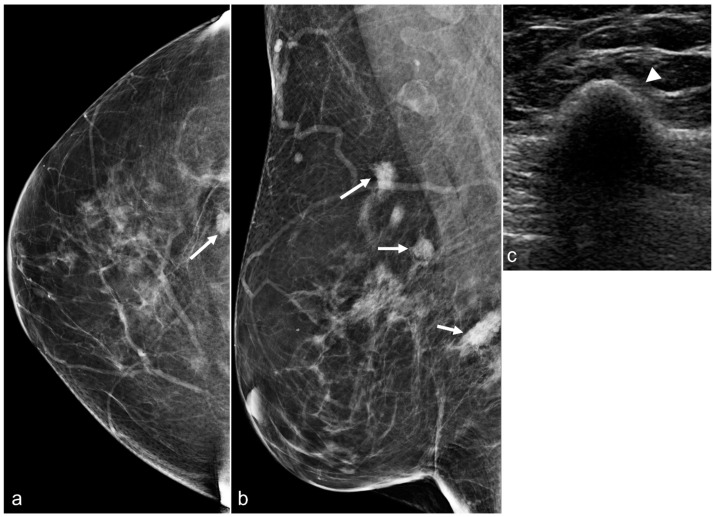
Mammograms of a 67-year-old woman with a history of retropectoral silicone implants and BES demonstrating several irregular dense breast masses (arrows) in the right breast on the CC (a) and the MLO (b) views. An ultrasound in the transverse view (c) confirms the classic snowstorm appearance of free silicone (arrowhead).
